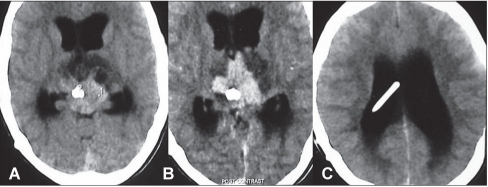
Patient 1: (A-C): This 8-year old boy presented with raised pressure, bilateral VIth nerve and upward gaze palsy of 8-months duration. His contrast enhanced CT scan showed a uniformly enhancing, infiltrative, posterior third ventricular lesion reaching the anterior third ventricle nearly until the foramen of Monro and causing hydrocephalus. The right ventriculoperitoneal shunt initially placed at another center got blocked and required revision.
